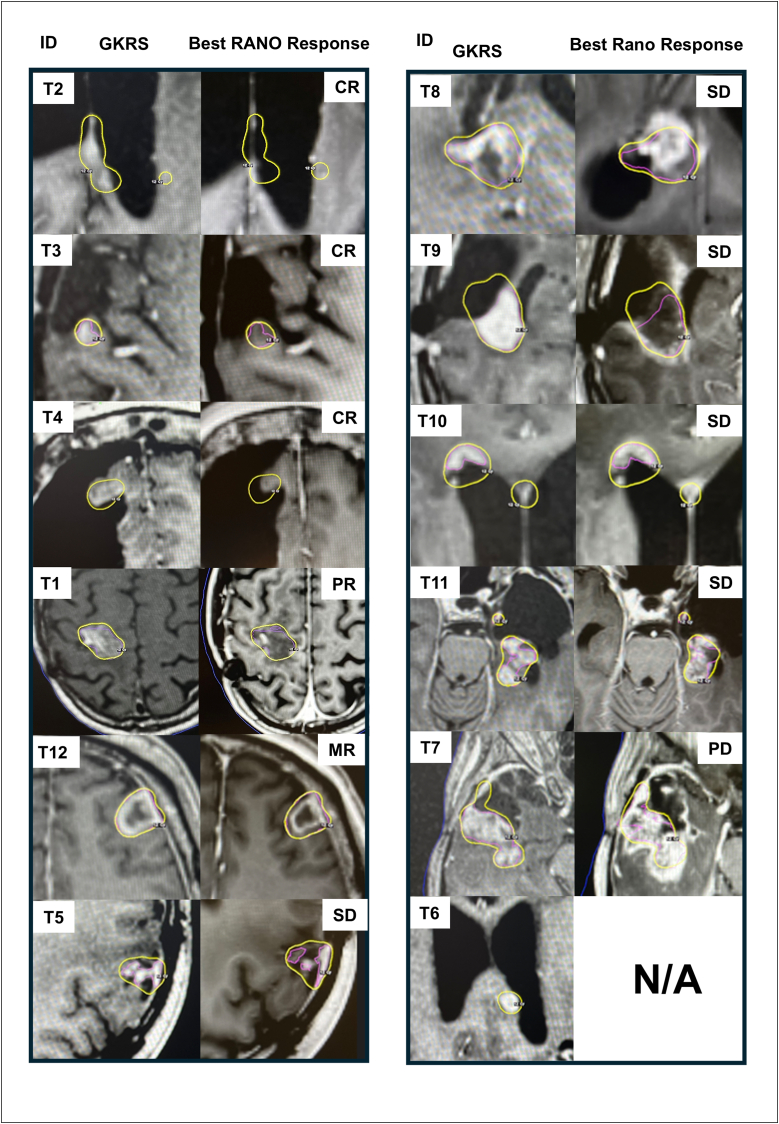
MRI at baseline and best RANO response during follow-up for 12 trial participants T1-T12. GKRS, Gamma Knife radiosurgery; T, trial; RANO, Response Assessment in Neuro-Oncology; CR, Complete response; PR, Partial response; MR, Minimal response; SD, Stable disease, PR, Progressive disease.
